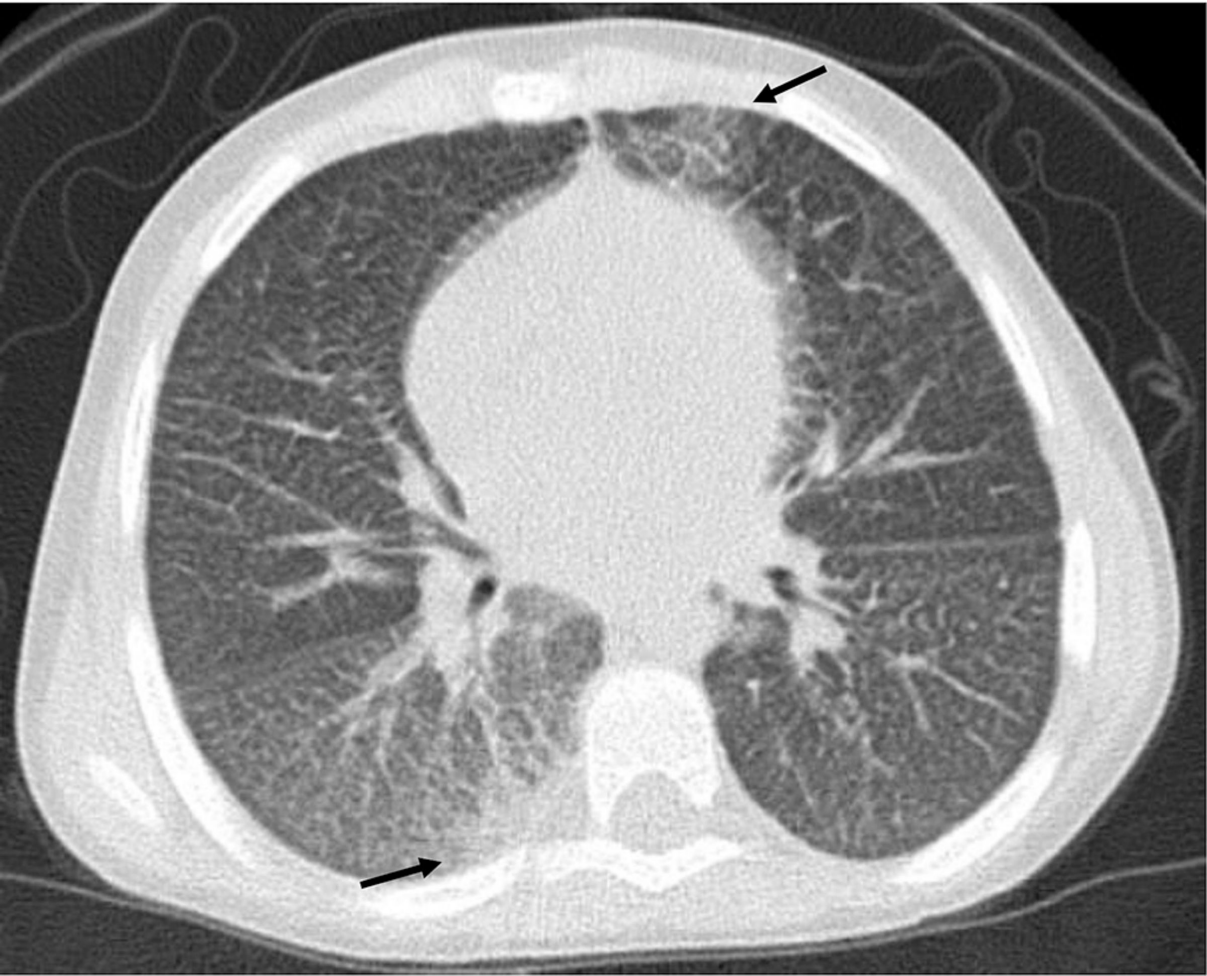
High resolution computed tomography scan of the chest from a 9-year-old
